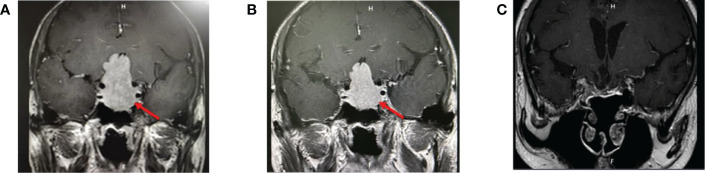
(A) Pituitary MRI before treatment: a large mass of equal T1, slightly longer T2 signal foci in the saddle area, clear boundaries, about 2.6 × 2.7 × 4.7 cm in size, protruding upward into the suprasellar cistern, semi-enveloping the internal carotid arteries on both sides, and the optic chiasm pressured upward. (B) Pituitary MRI after 6 months of octreotide: similar to that before treatment. (C) Pituitary MRI 9 months after surgery.
